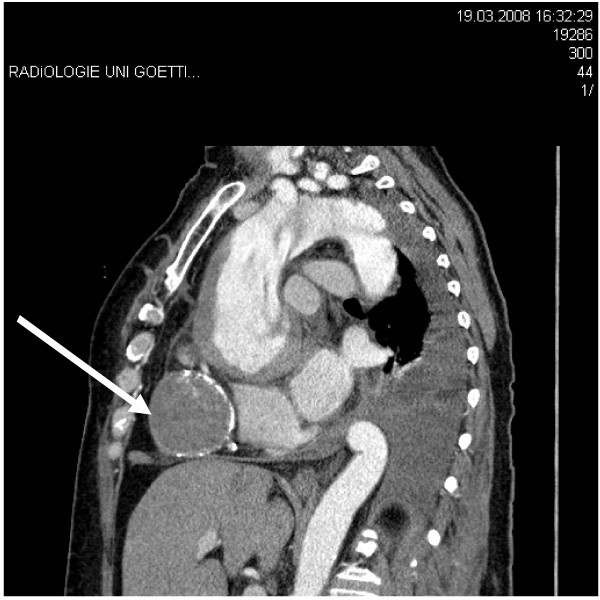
CT scan showing an adherent structure which compressing the right ventricle.
